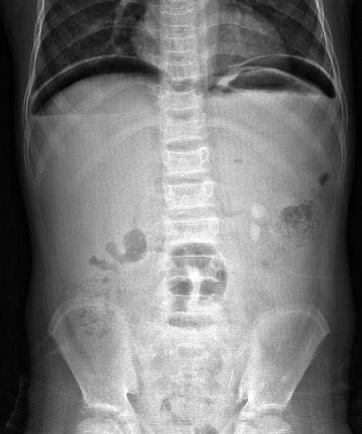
Free air beneath the diaphragm at abdominal x-ray film
